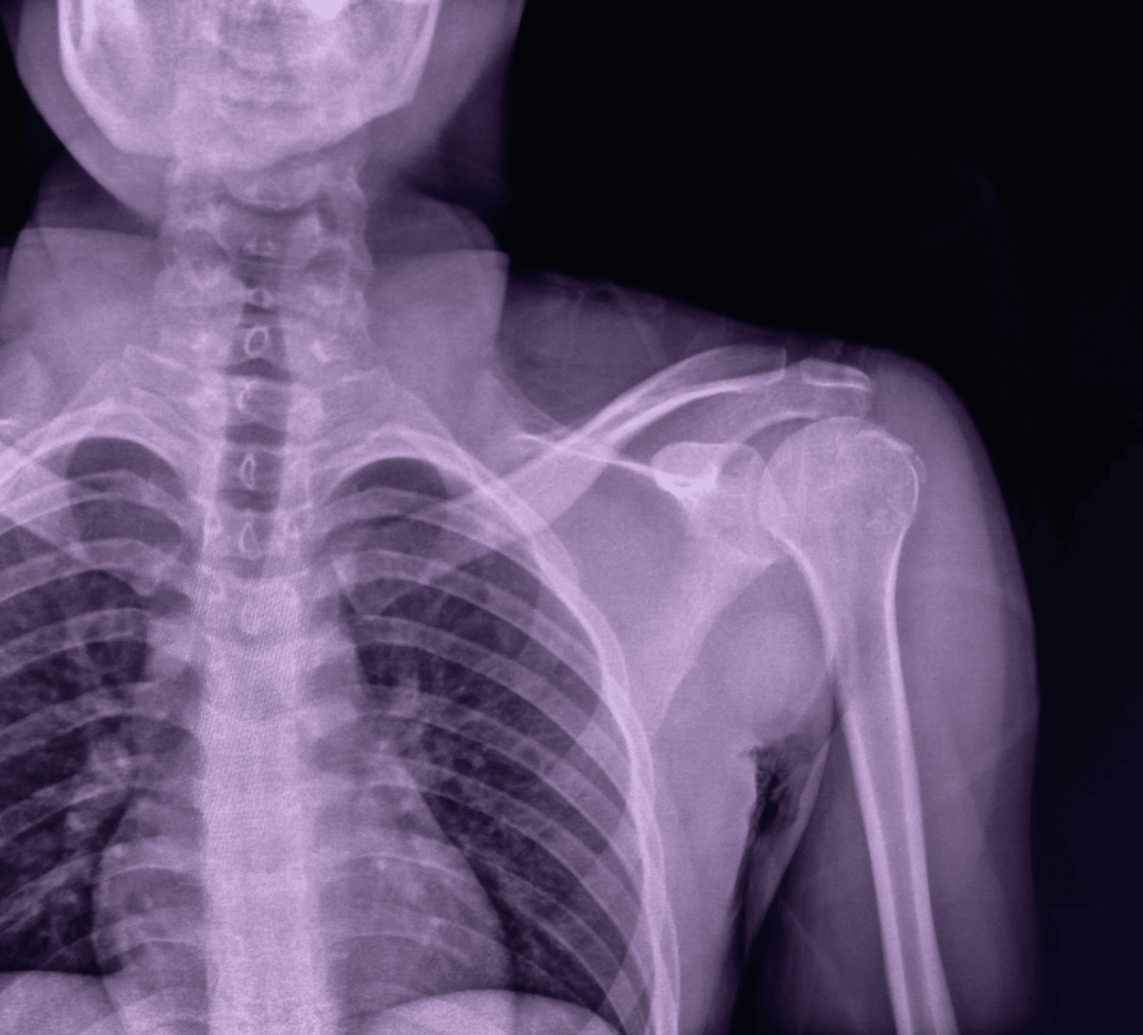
Post-reduction control X-ray showing the correct positioning of the humeral head relative to the glenoid cavity
